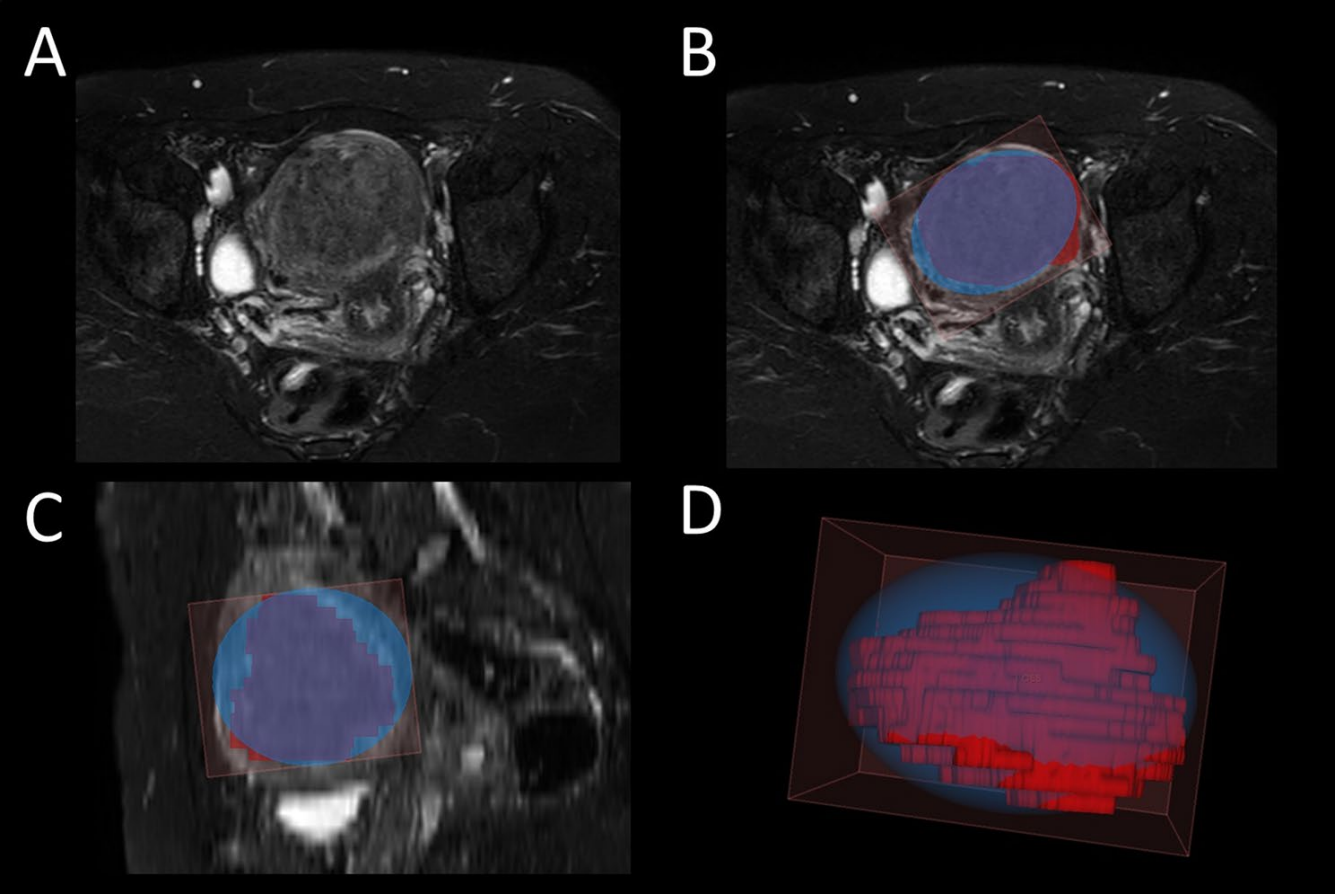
Overestimation of the volume of uterine fibroid by using the maximal diameters and the ellipsoid formula compared to the actual segmented volume. The axial reconstruction of the T2W SPAIR MRI sequence of a patient with multiple uterine fibroids (A). The manual segmentation of the largest fibroid (red) and its bounding ellipsoid (blue) on the axial plane (B), sagittal plane (C), and in three-dimensional reconstruction (D)
